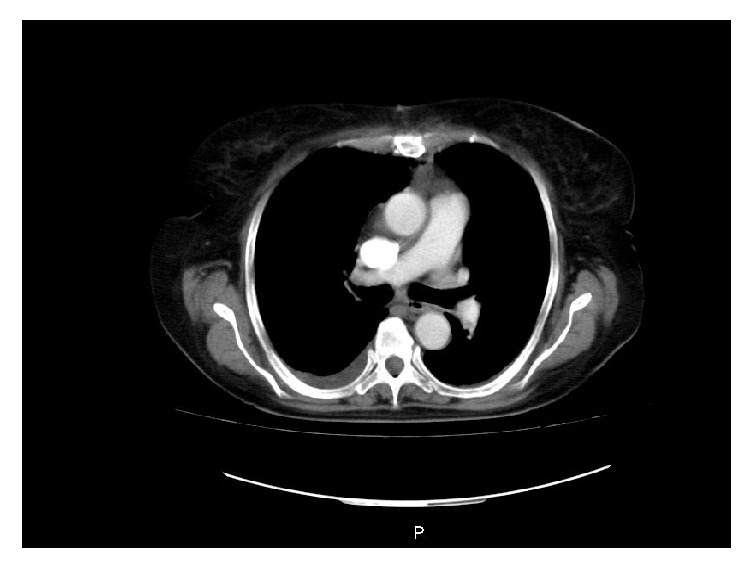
CT pulmonary angiography showing the bifurcation of the right pulmonary artery without clot.
